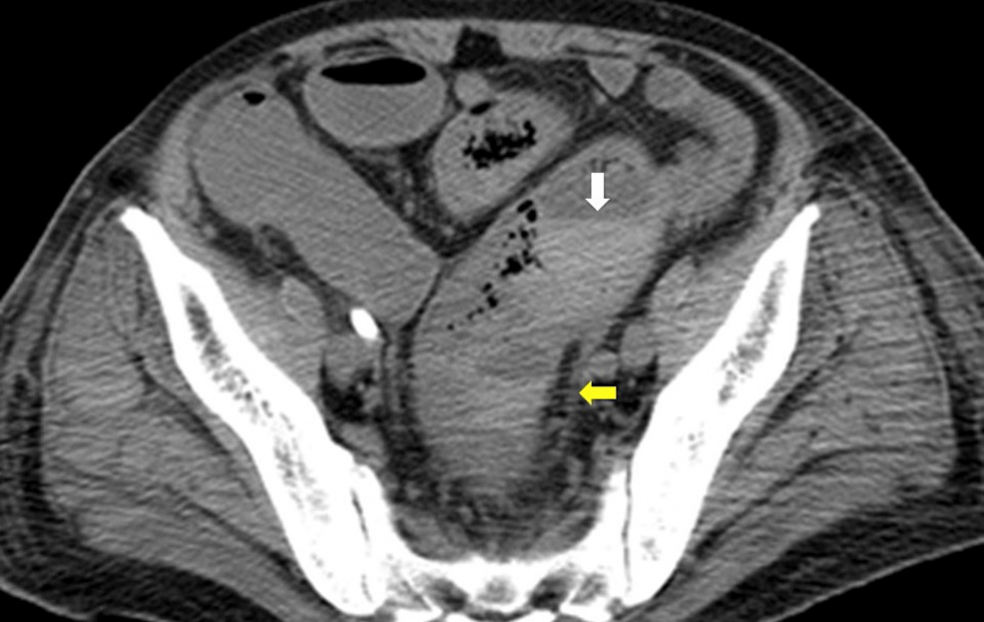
Abdominal computed tomography images Intramural emphysema was observed in the sigmoid colon (yellow arrow) and very bright intestinal fluid was observed (white arrow).
